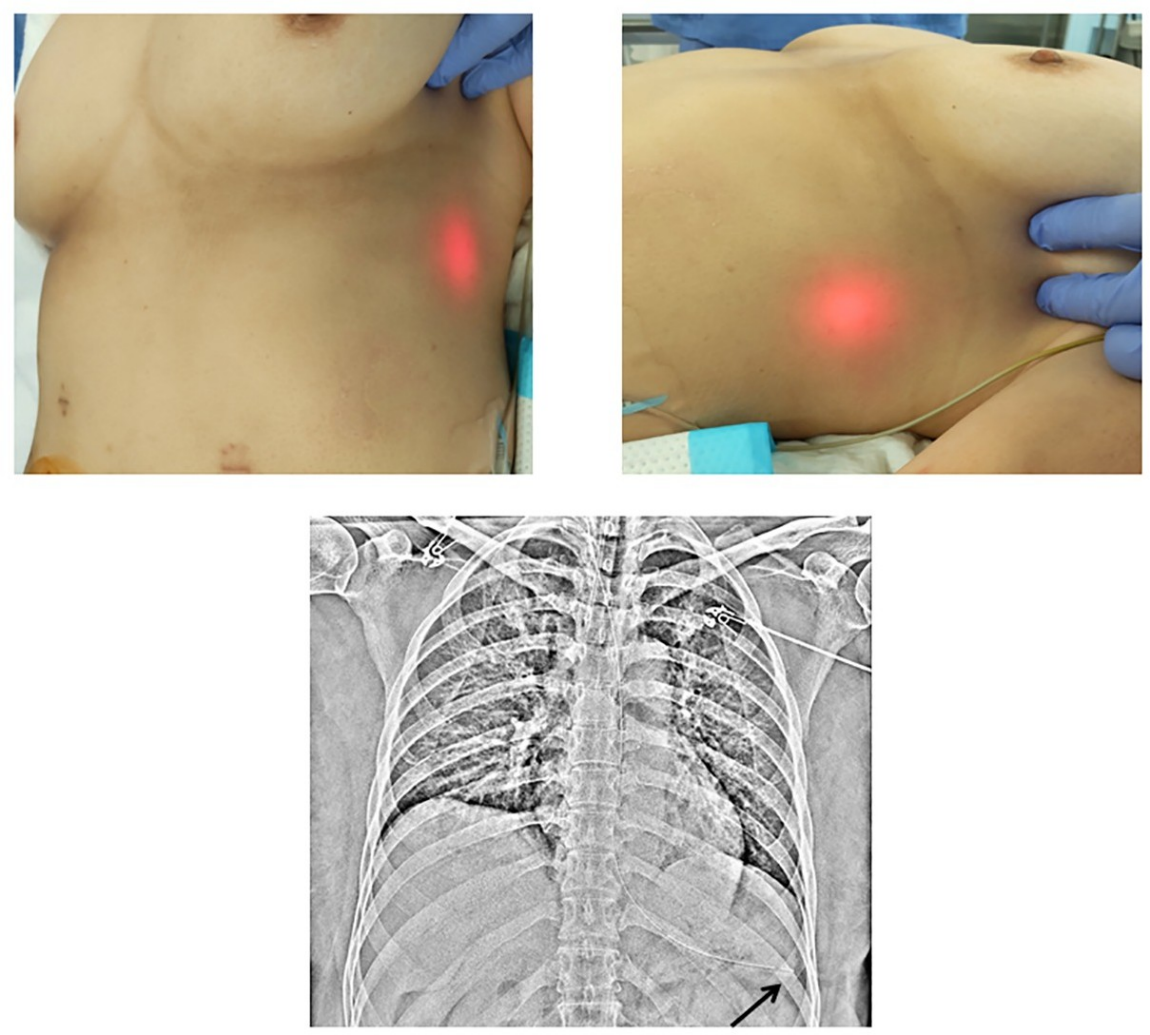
Positive Biologically transparent light sign in the lateral epigastric area and corresponding X-ray image. The arrow indicates the tip of the nasogastric tube.
